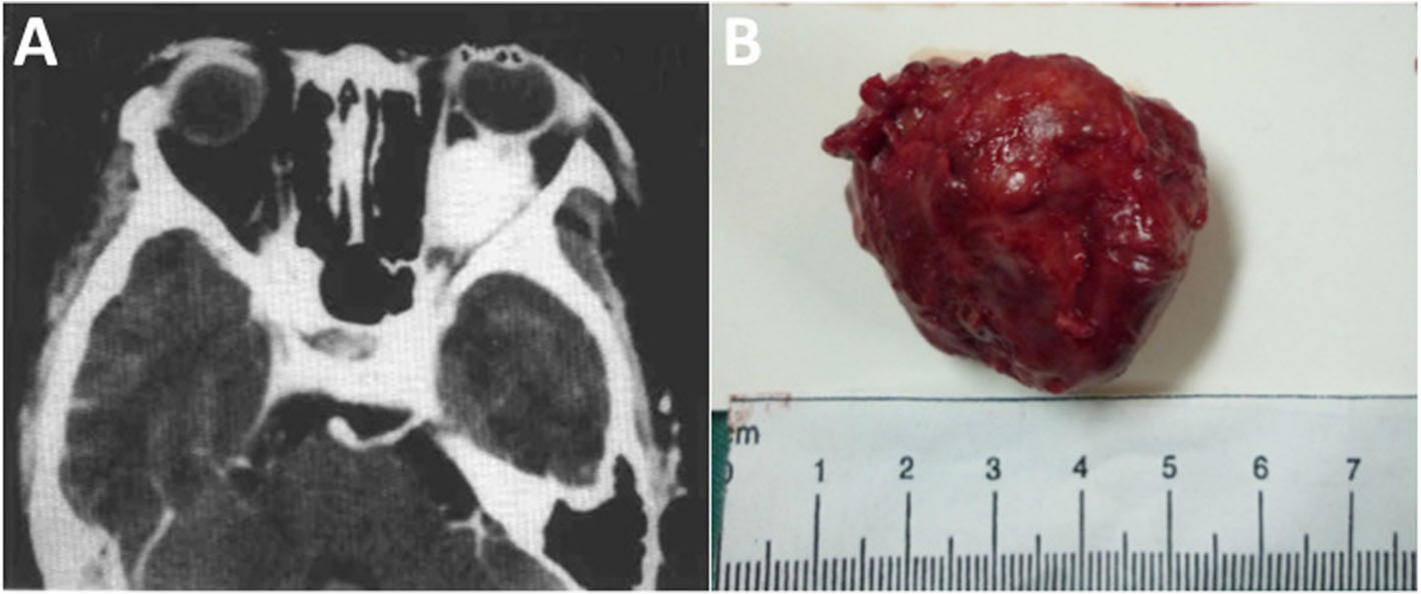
a Axial CT scan of the orbits in patient 1, showing a heterogeneous mass surrounding the optic nerve in the posteroinferior orbit. b Left orbital mass resected from patient 2
